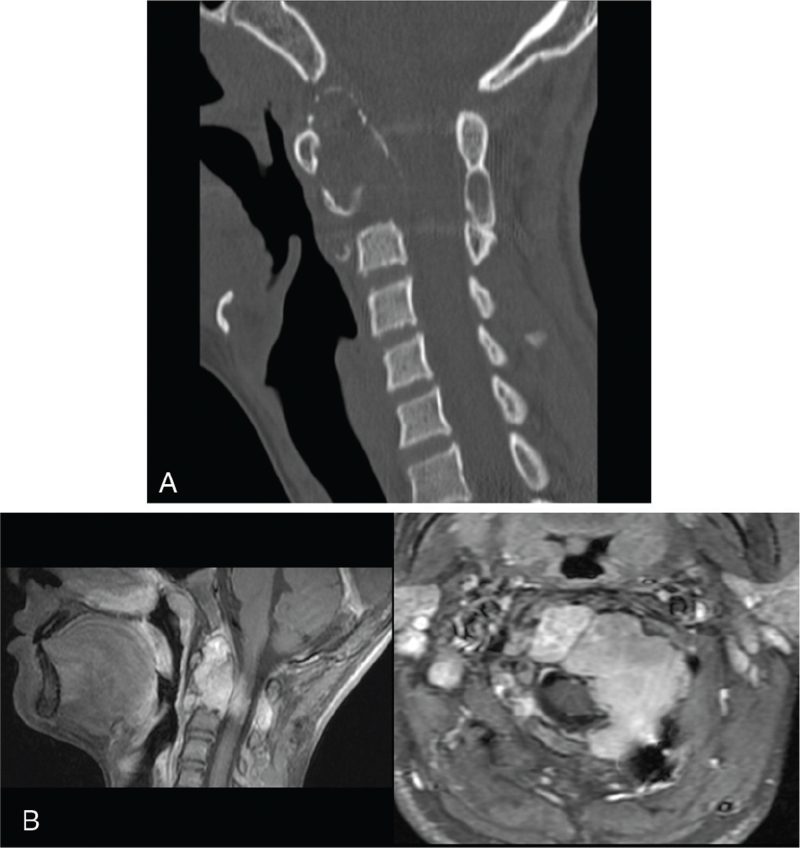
Computed tomography (A) and magnetic resonance (B) images of the cervical spine 1 yr after the surgery. Magnetic resonance images revealed gradual resorption of the transplanted bone and recurrent tumors.
